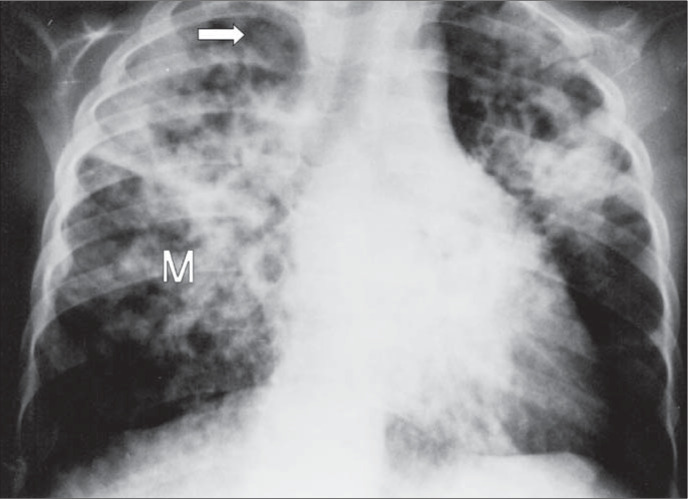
Chest x-ray upon admition. M = miliary pattern. Arrow shows cavities at the right upper lobe.
